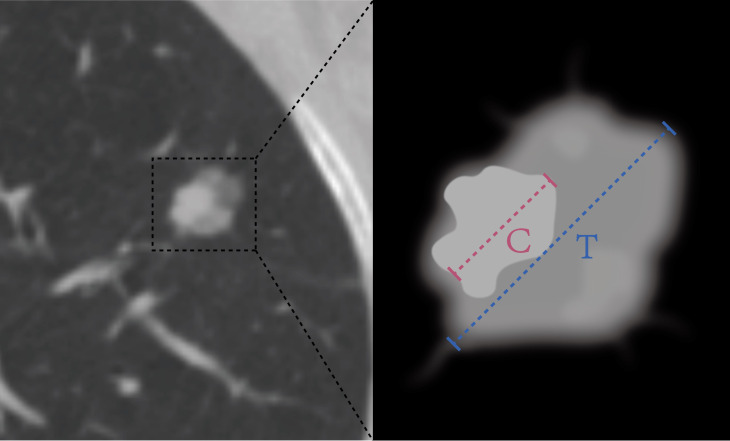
The HRCT imaging information measurement contains the following: (1) the diameter of the tumor (T) was defined as the largest axial diameter of the lesion on the lung window setting (Blue dotted line); (2) the diameter of consolidation (C) on the axial image on the lung window setting was measured, and consolidation was defined as an area of increased opacification that completely obscured the underlying bronchial structures and vascular markings (Pink dotted line); (3) the ratio of the maximum diameter of consolidation relative to the maximum tumor diameter from the lung window (CTR).
